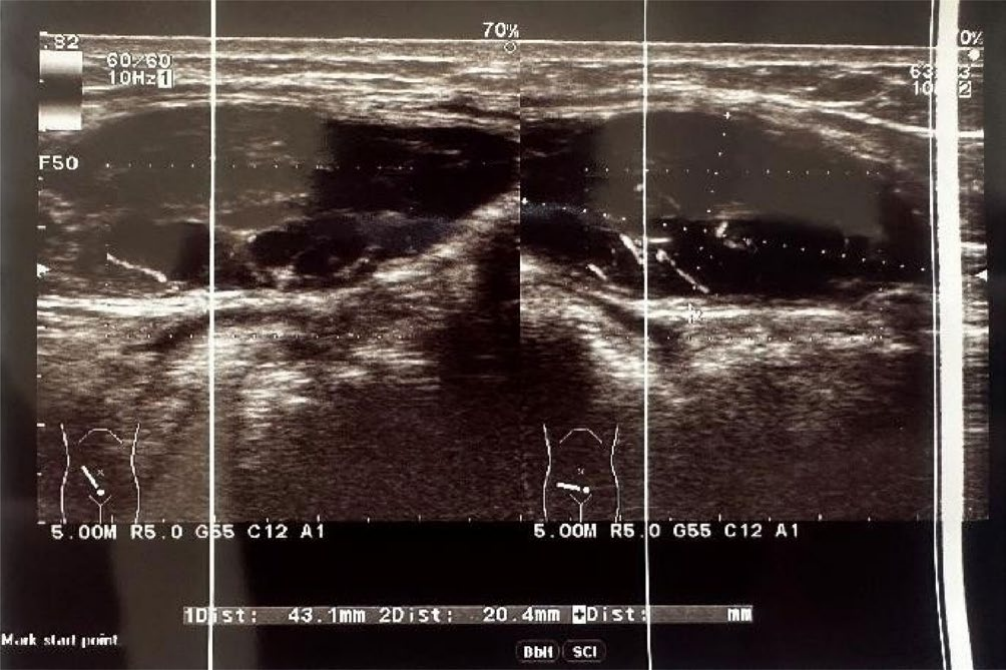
Ultrasonography of the abdominal wall showing multiple hypoechoic, disc‐shaped lesions.
